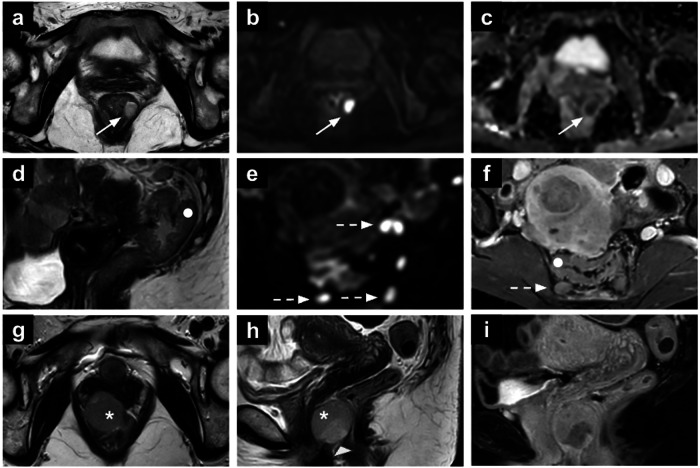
Rectal abscess (a–c). Axial T2-WI (a), high *b*-value DWI (b) and the ADC map (c) of a 45-year-old patient underwent MRI for endometrial cancer staging revealing an intersphincteric abscess in the upper third of the left anal canal (arrows). Ulcerative colitis (d–f). Sagittal T2-WI (d), axial DWI (e) and post-contrast T1-WI (f) showing incidental ulcerative colitis, characterised by diffuse rectal wall thickening with increased enhancement (white dot in d–f). Multiple reactive mesorectal nodes are also noted (dotted arrows in e, f). GIST (g–i). Axial T2-WI (g), sagittal T2-WI (h) and post-contrast T1-WI (i) in a 51-year-old patient undergoing MRI for adnexal mass characterisation. The images show a round-shaped mass in the recto-vaginal septum (arrowhead), displacing the vagina anteriorly and the rectum posteriorly. Two components can be recognised: a solid component (asterisk) showing intermediate SI on T2-WI, and a necrotic component (arrowhead in h) showing high SI on T2-WI and lack of enhancement. SI, signal intensity; WI, weighted images
